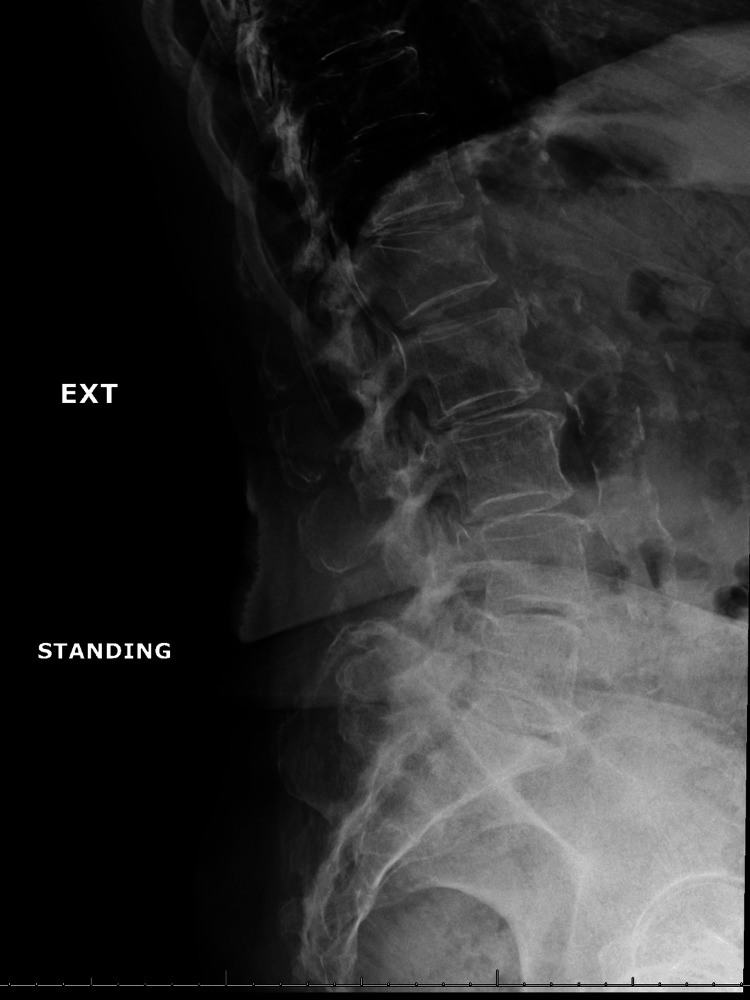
Lumbosacral X-ray extension view showing grade 1 retrolisthesis of L1 on L2 and L2 on L3.
